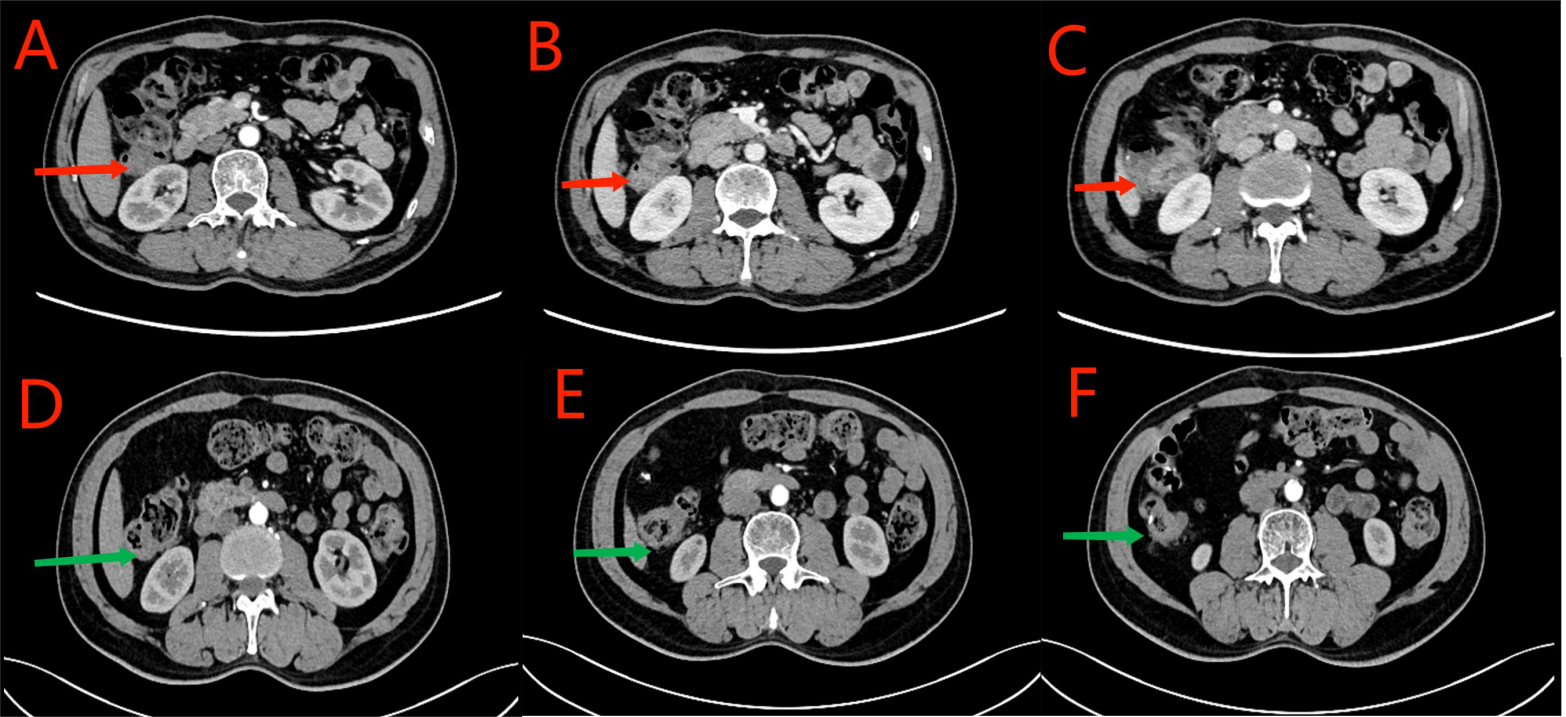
(A–C) CT scan conducted on May 19, 2023. Red arrows indicate segmental thickening of the anastomosis and distal intestinal wall. The tumor was smaller in size and less dense than that observed previously. Lymph nodes in the surrounding adipose space are shown; they were smaller in size than those observed previously. (D–F) CT scan conducted on July 21, 2023. Green arrows indicate local thickening of the anastomosis and distal intestinal wall, which was significantly reduced than that observed previously, and small lymph nodes in the surrounding adipose space.
